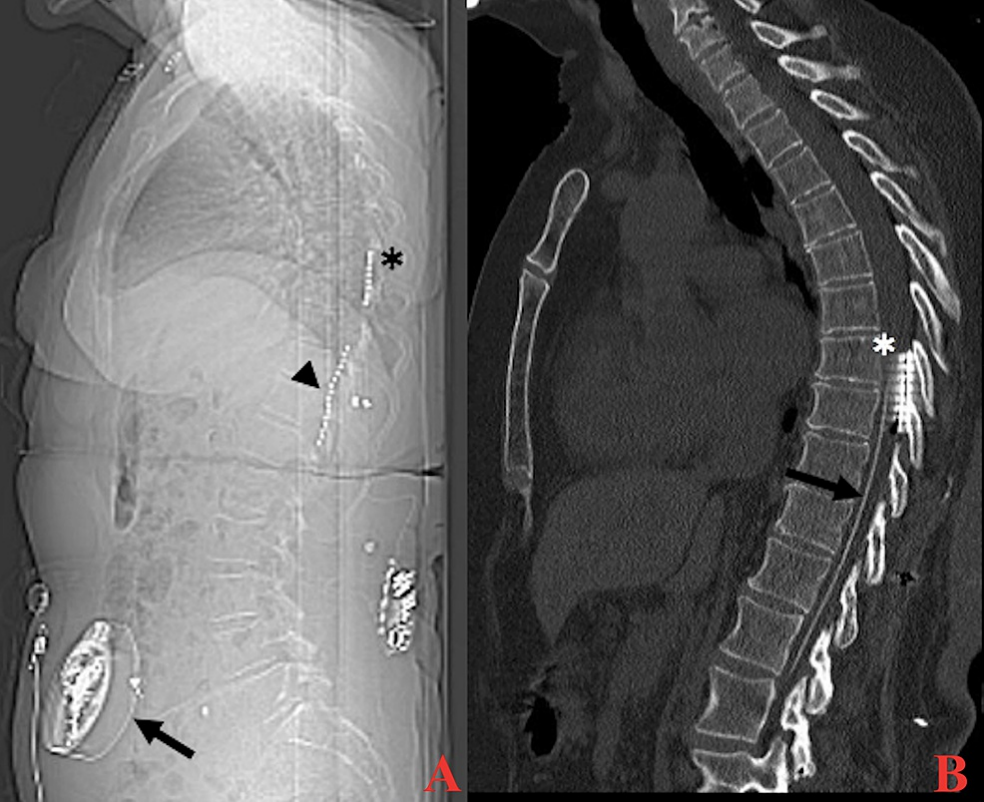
Lateral x-ray (A) and sagittal computed tomography of the thoracic spine (B) demonstrating the intrathecal analgesia pump reservoir and intra-dural catheter (black arrow), single percutaneous electrode (arrowhead), and paddle electrode (asterix).
